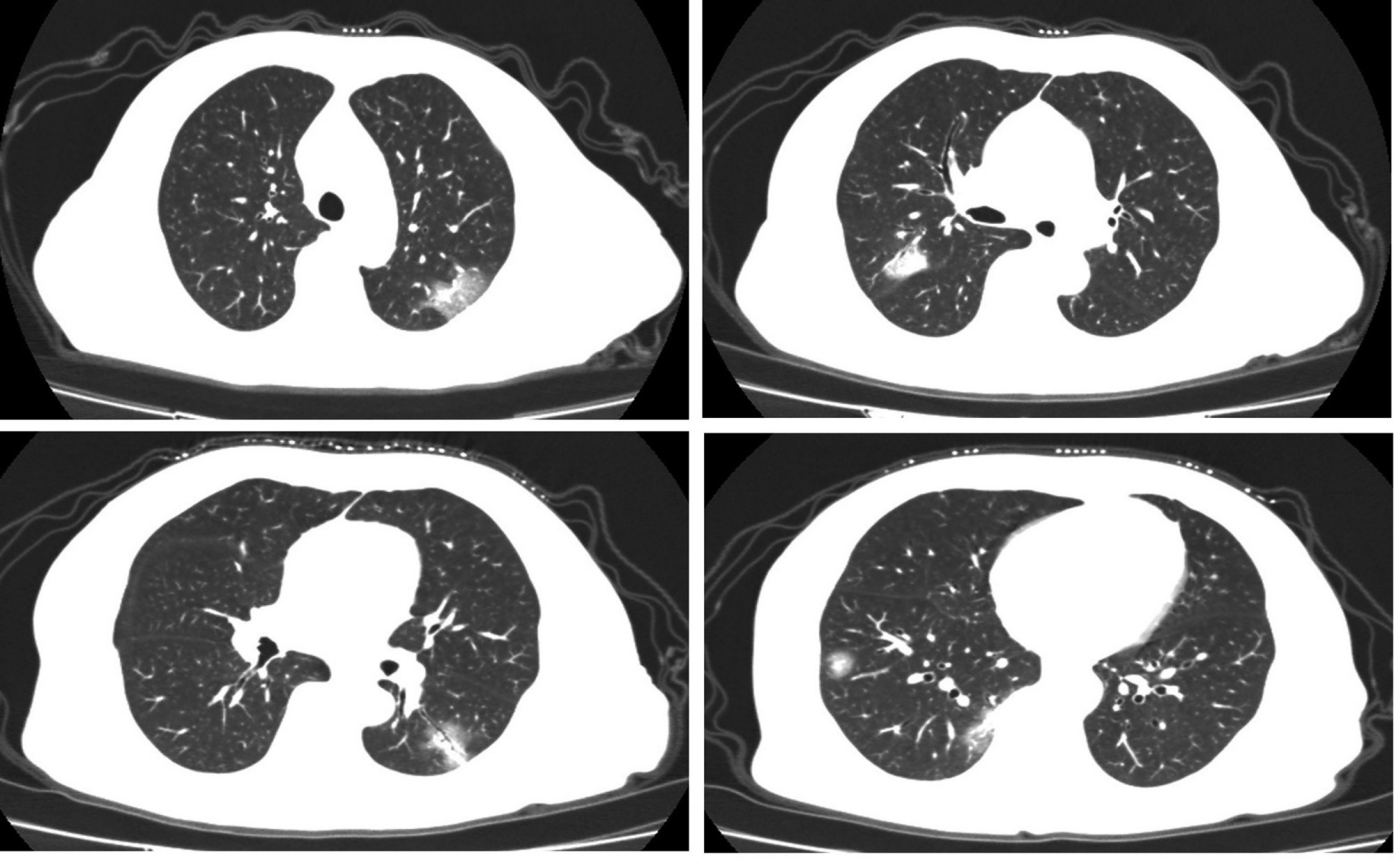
Case 1: CT chest result showed multiple peripheral solid and ground-glass images on bilateral lungs.
